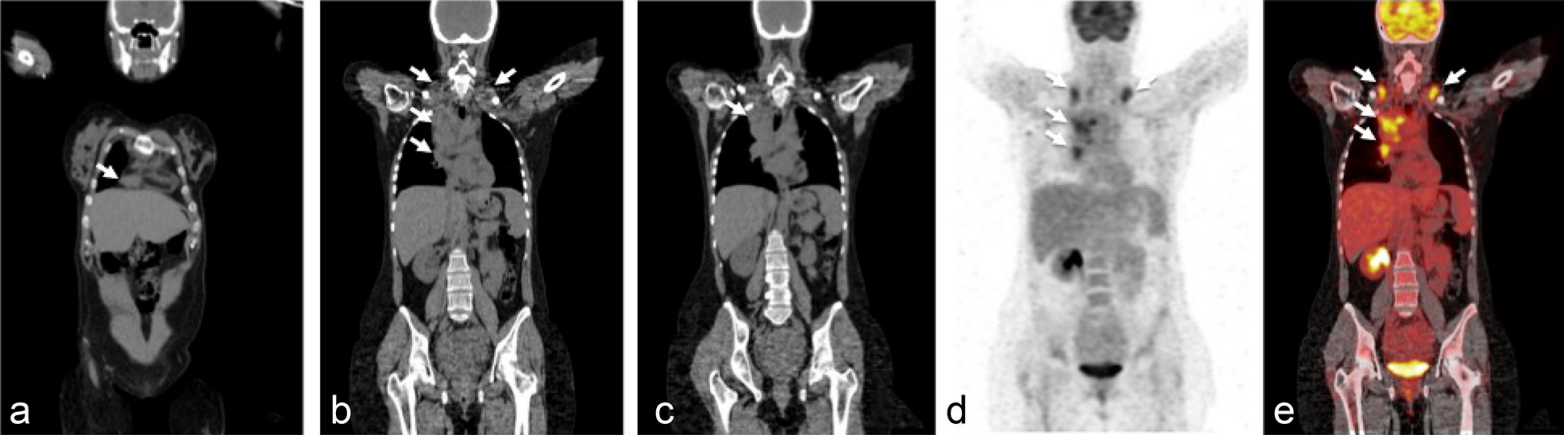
Images from scanning performed 2 weeks after delivery. Fludeoxyglucose-positron emission tomography/CT images (a–e) showing multiple areas of increased uptake in cardiophrenic, bilateral supraclavicular and mediastinal lymph nodes (a–e; arrows).
